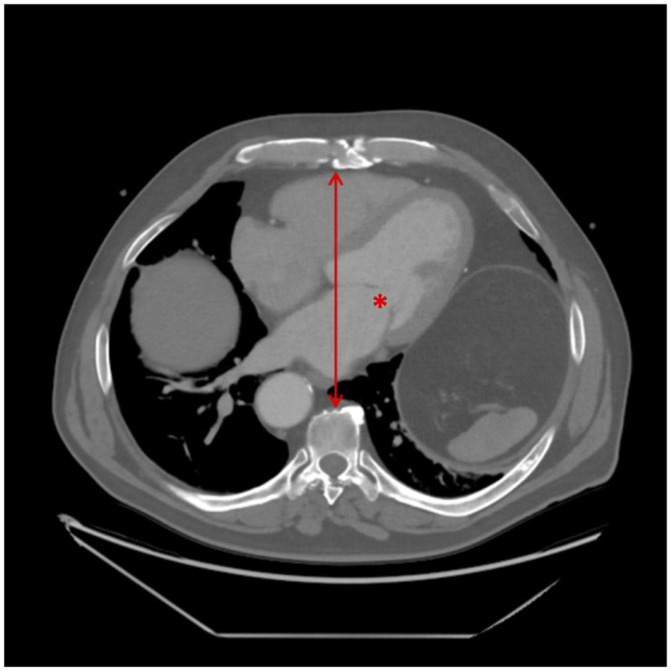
Measurement of anteroposterior (AP) diameter on preoperative CT imaging. The asterisk (*) indicates the mitral valve at the approximate level of A2/P2. The line with arrowheads represents the anteroposterior distance, measured from the posterior aspect of the sternum to the anterior surface of the corresponding vertebral body.
